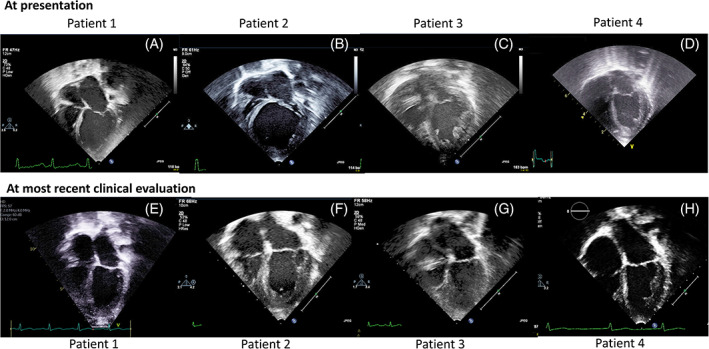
Echocardiogram apical four‐chamber view at presentation (A–D) and latest follow‐up (E–H) for patients 1–4 who have had sustained resolution of heart failure and improvement of cardiac function. Improvement in left ventricular (LV) size is evident in all patients. LV dilation is most evident in (B) and noncompaction most evident in (C)
